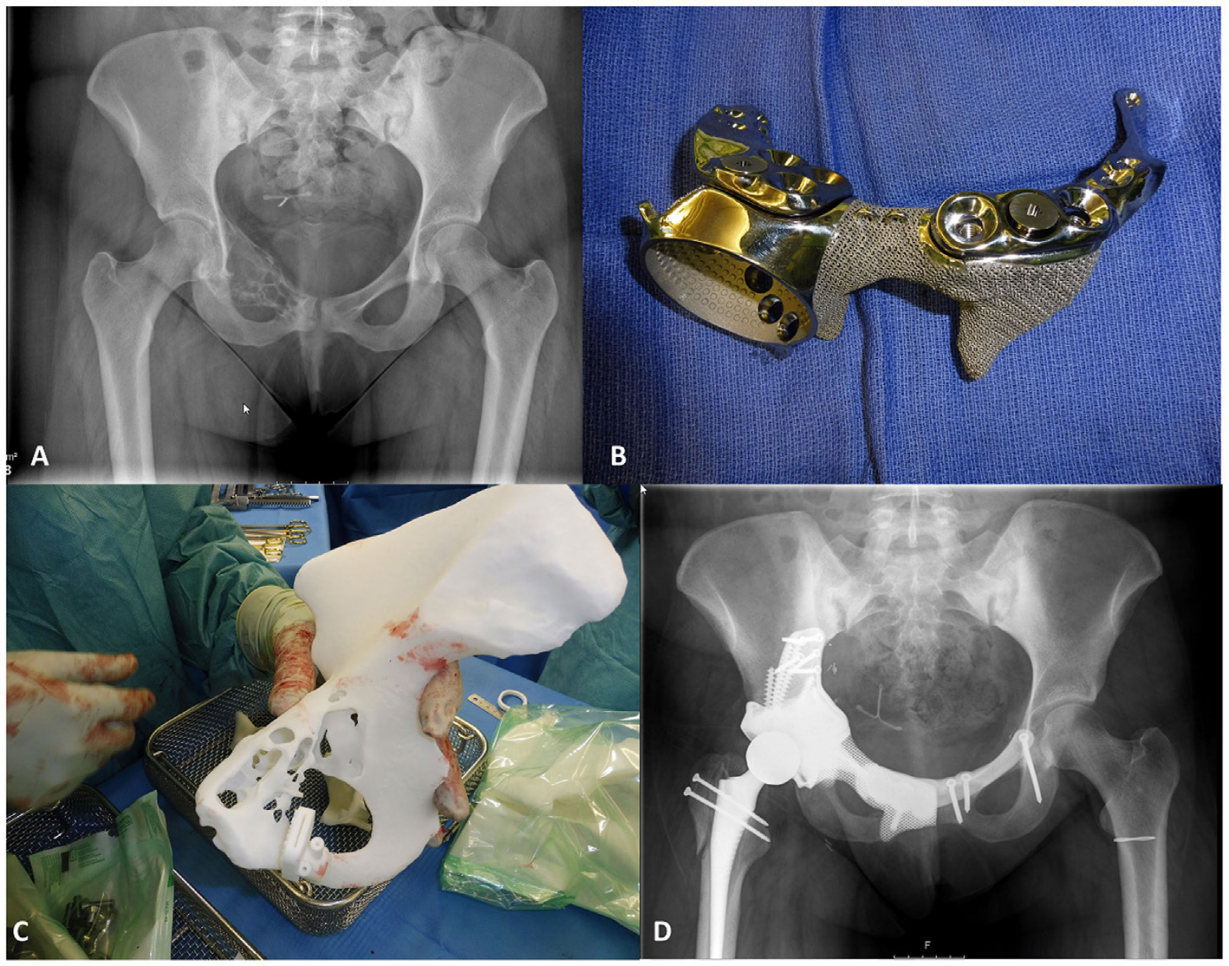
Patient-specific instrumentation for pelvic tumor resection surgery. These images show the application of patient-specific instrumentation for pelvic tumor treatment. Implant and template manufactured by Mobelife NV, Leuven, Belgium. (A) A pre-operative X-ray radiograph; (B) the implant; (C) the patient-specific guide; and (D) a post-operative X-ray radiograph (Courtesy of Prof. Dr. K Siebenrock, Inselspital, University of Bern, Switzerland).
